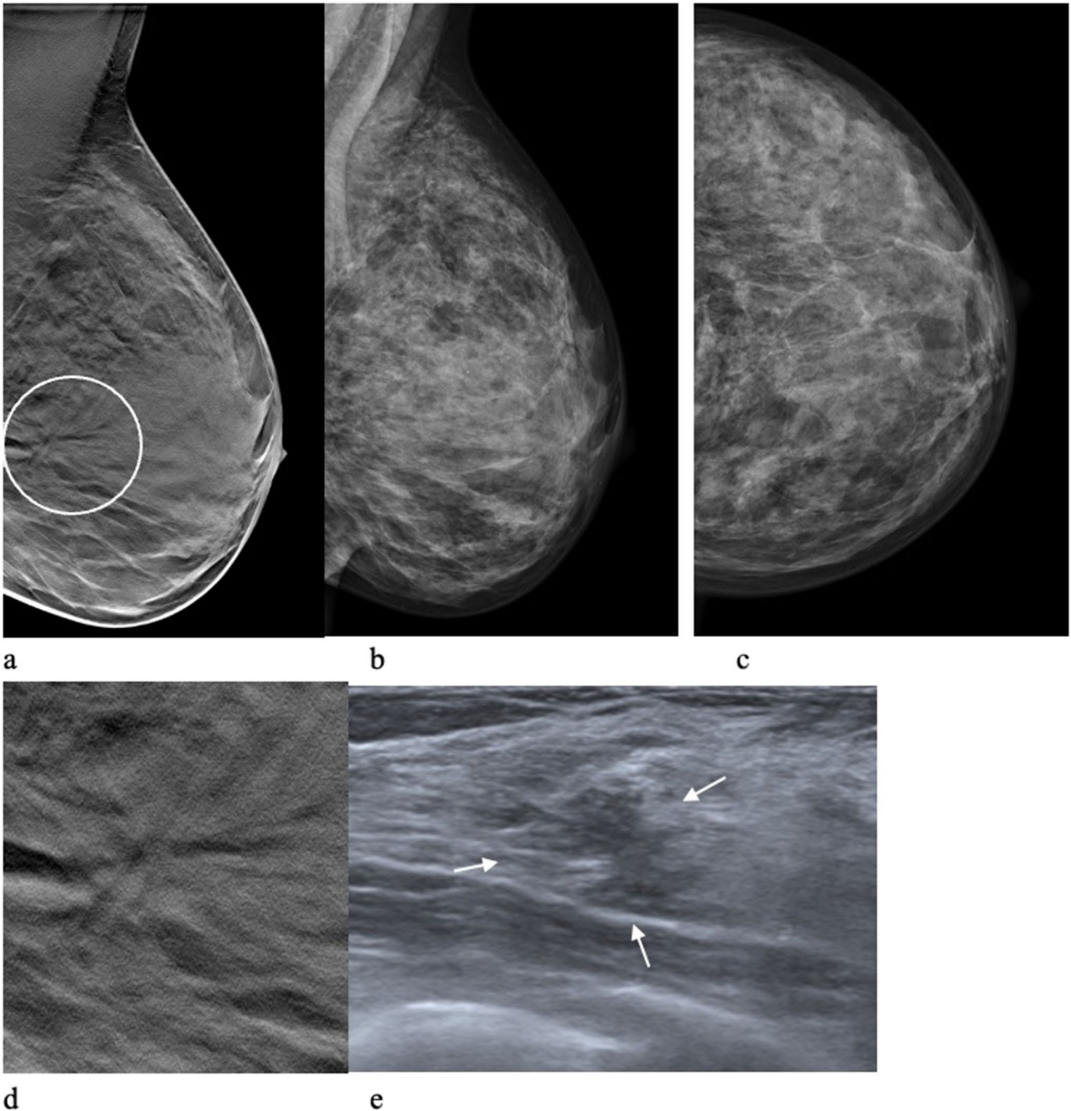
A 48-year-old woman who was recalled in the DBT group due to a stellate distortion (circle). One-view digital breast tomosynthesis (a) and mediolateral oblique (b) and craniocaudal (c) views at digital mammography and at screening. The false-positive finding enlarged (d). Ultrasound at work-up (e) showed a diffuse irregular lesion (arrows). Core needle biopsy confirmed a radial scar
